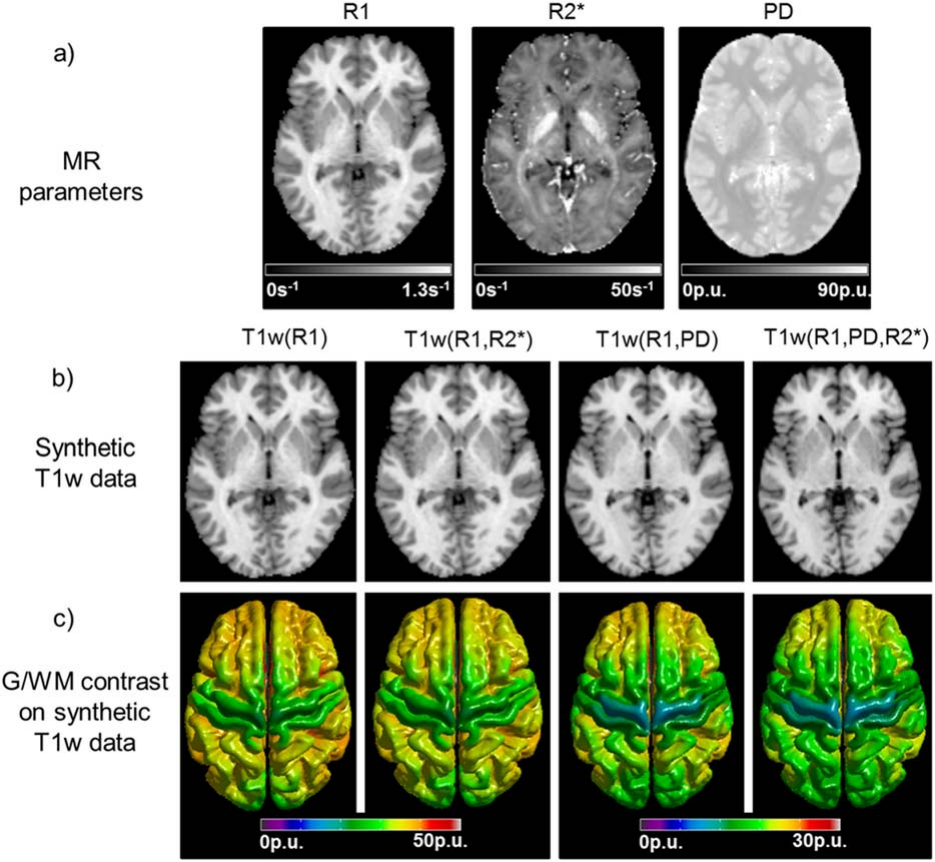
Example of synthetic MPRAGE T1w images. Individual quantitative maps of the MRI parameters R1, PD and R2* (a). Synthetic T1w MPRAGE images computed from the maps of MRI parameters and the MPRAGE signal equation [Eq. (1)] (b). Gray‐white matter contrast in the synthetic T1w images calculated from Eq. (2) (c). [Color figure can be viewed in the online issue, which is available at http://wileyonlinelibrary.com.]
